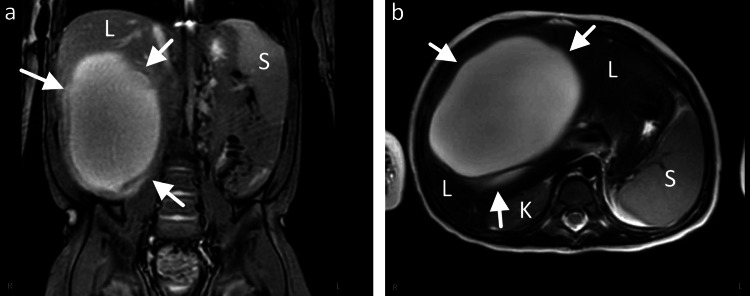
MRI abdomen coronal (a) and axial (b) views of 13.7 x 13.6 x 11.5 cm mixed solid and predominately cystic lesion within the right inferior hepatic lobe L: normal liver; S: spleen; K: kidney; arrows: boundaries of hepatic neoplasm
